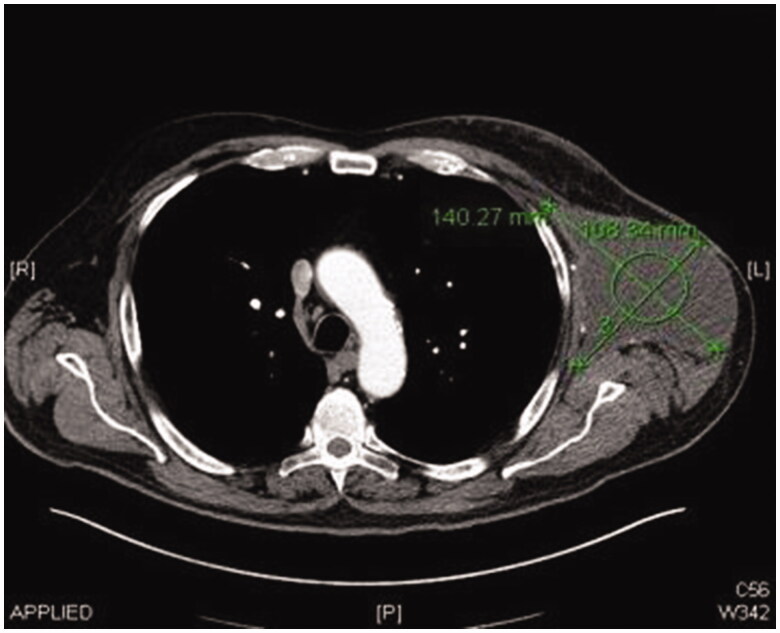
Preoperative CT scan image showing left axillary chronic seroma.
